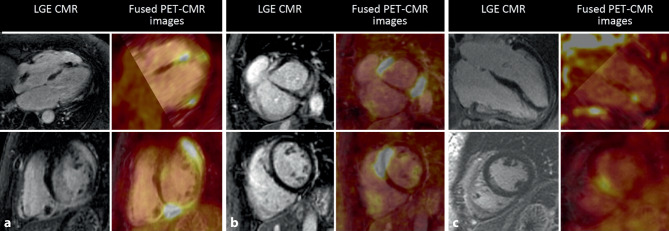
Examples of late gadolinium enhancement (LGE) images and ^18^F‑fluorodeoxyglucose (^18^F‑FDG) positron emission tomography (PET) images with myocardial uptake in 3 patients diagnosed with cardiac sarcoidosis. a Extensive mid-myocardial LGE and ^18^F‑FDG uptake inferior and epicardial to mid-wall LGE apicoseptal in 4‑chamber view. Transmural LGE basal anterolateral and inferior with involvement of right ventricle in short-axis view. b Mid-myocardial LGE basal inferolateral corresponding to location of ^18^F‑FDG uptake on PET scan. c Focal to subendocardial LGE midventricular septum corresponding to location of ^18^F‑FDG uptake on PET scan in short-axis and 4‑chamber views. *CMR* cardiovascular magnetic resonance imaging
